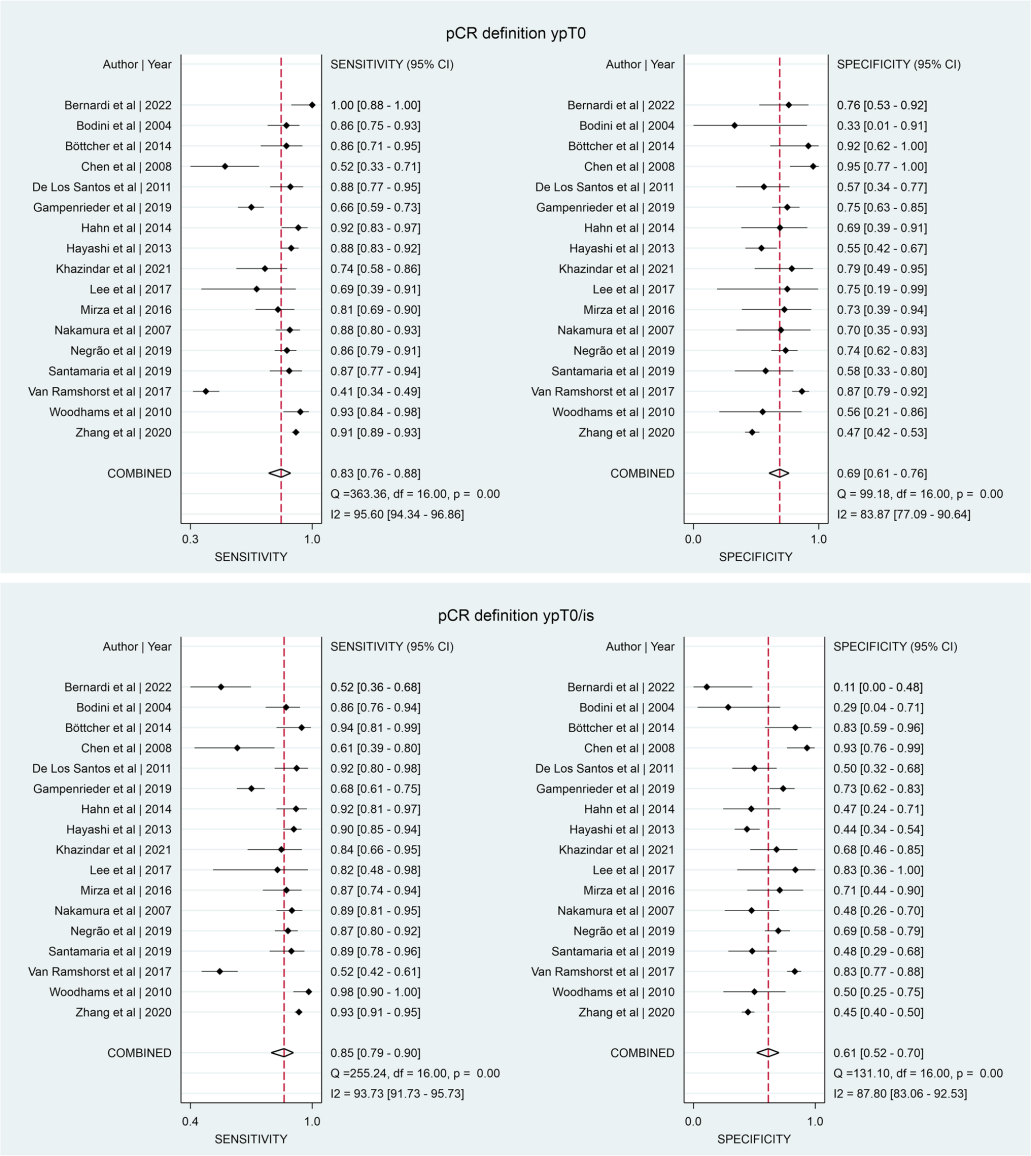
Pooled sensitivity and specificity for detection of residual disease by MRI between pCR defined as ypT0 and ypT0/is
